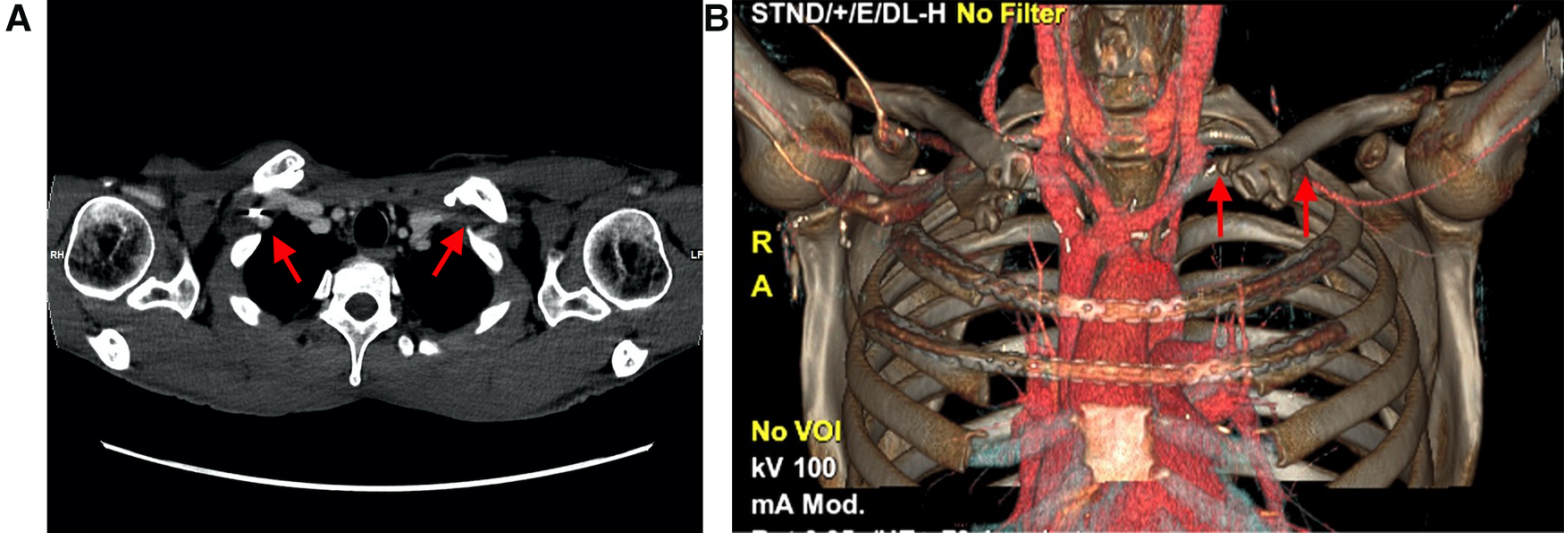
(A) Computed tomography revealed more narrowing at the level of the vein on the left side (5 mm) than the right side (11 mm). (B) Three-dimensional reconstruction indicating loss of contrast over the subclavian vein due to delayed opacification, secondary to stenosis.
